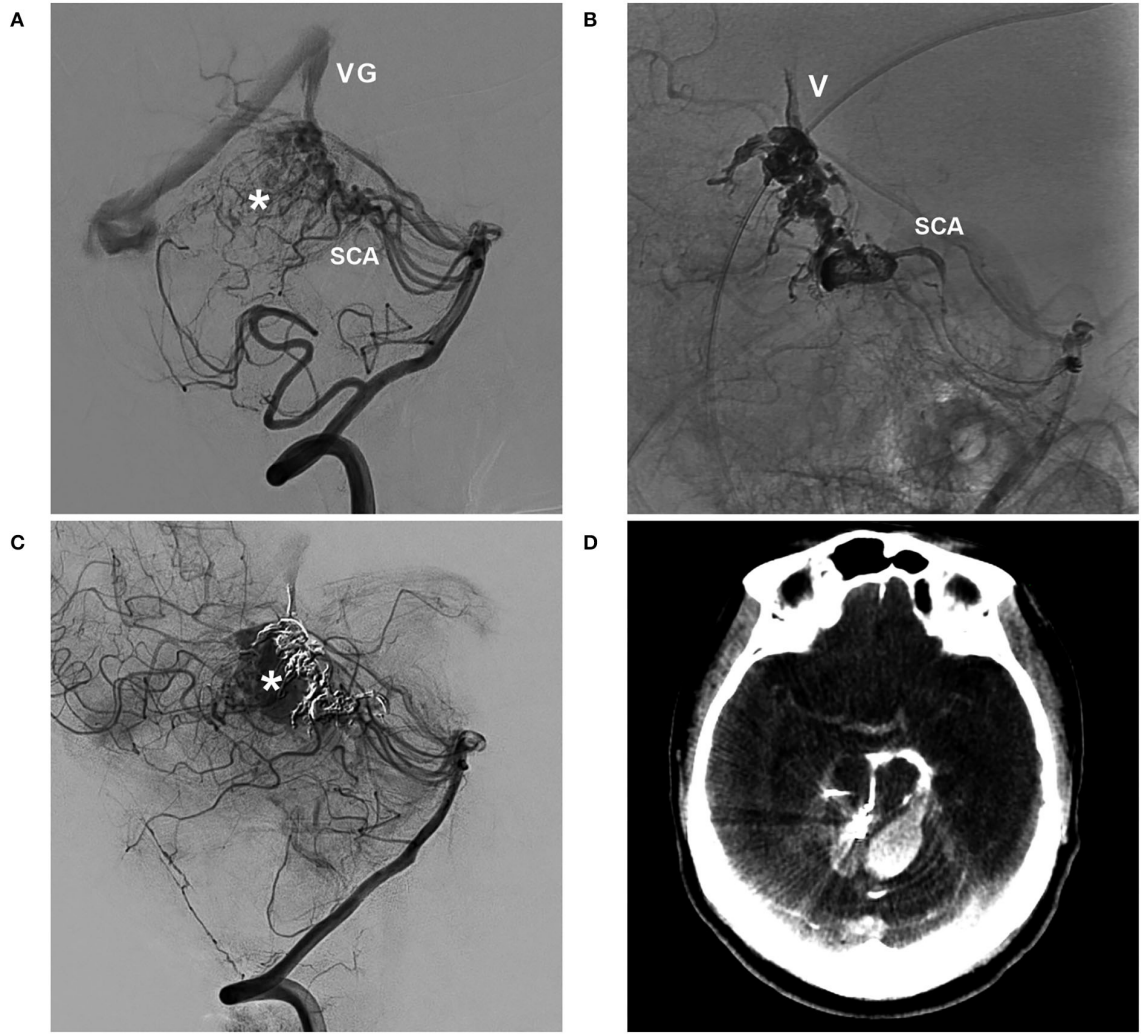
BAVM with intraoperative rupture of area of perinidal angiogenesis (case 1). (A) Arterial-phase angiogram of the VA showing the BAVM supplied by the SCA, which drains into the VG; the asterisk indicates perinidal angiogenesis. (B) Unsubtracted angiogram of the VA showing Onyx casting in the SCA, nidus, and draining vein (V). (C) Angiogram of the VA showing contrast agent extravasation from intraoperative rupture of the area of perinidal angiogenesis (asterisk). (D) X-per CT showing contrast agent extravasation. BAVM, brain arteriovenous malformation; CT, computed tomography; SCA, superior cerebellar artery; VA, vertebral artery; VG, vein of Galen.
